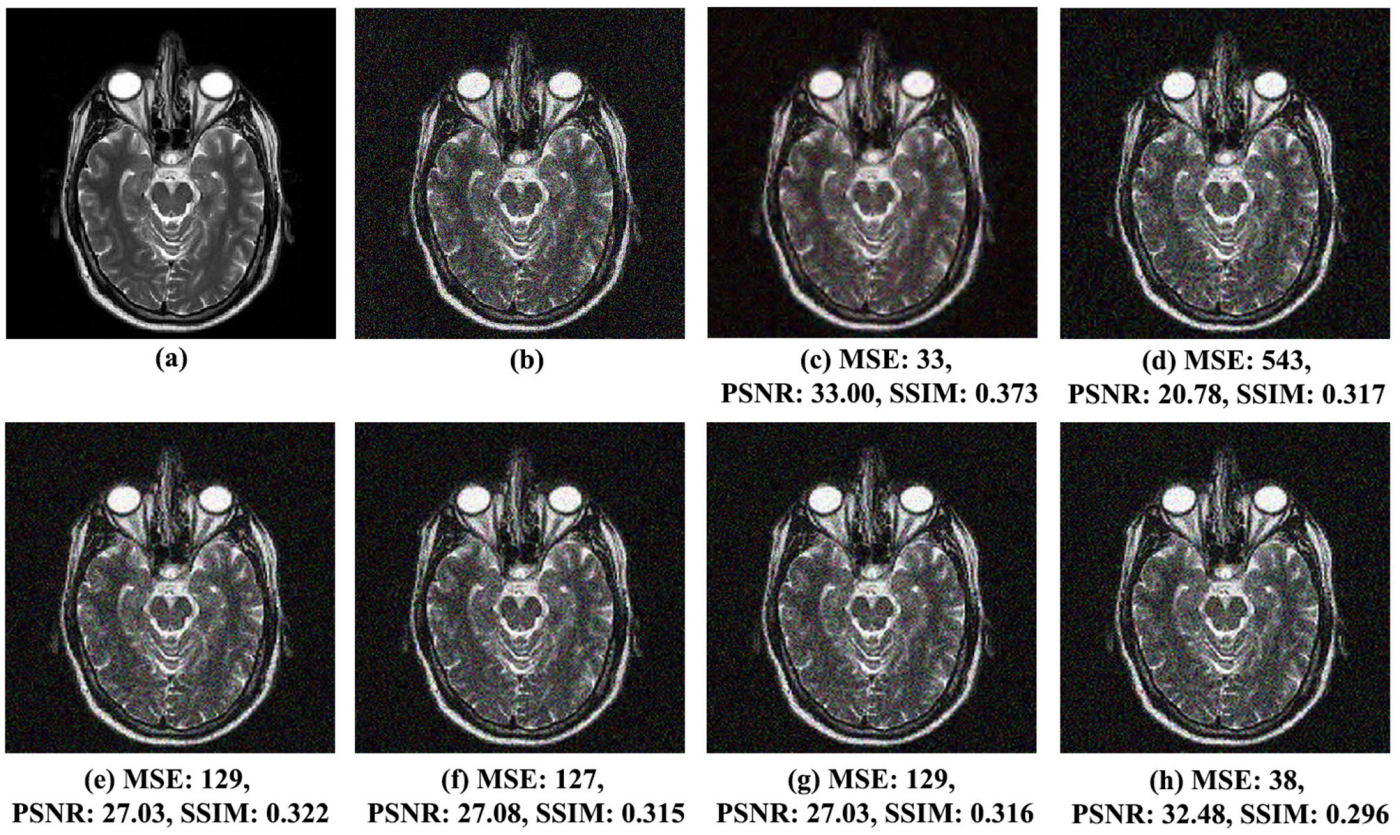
Comparison of noise reduction performance for MRI Brain Image 2 with noise variance of 0.03: (A) original image, (B) noisy image, (C) proposed method, (D) VisuShrink, (E) BayesShrink, (F) Gaussian Kernel, (G) improved threshold, and (H) AGGD.
